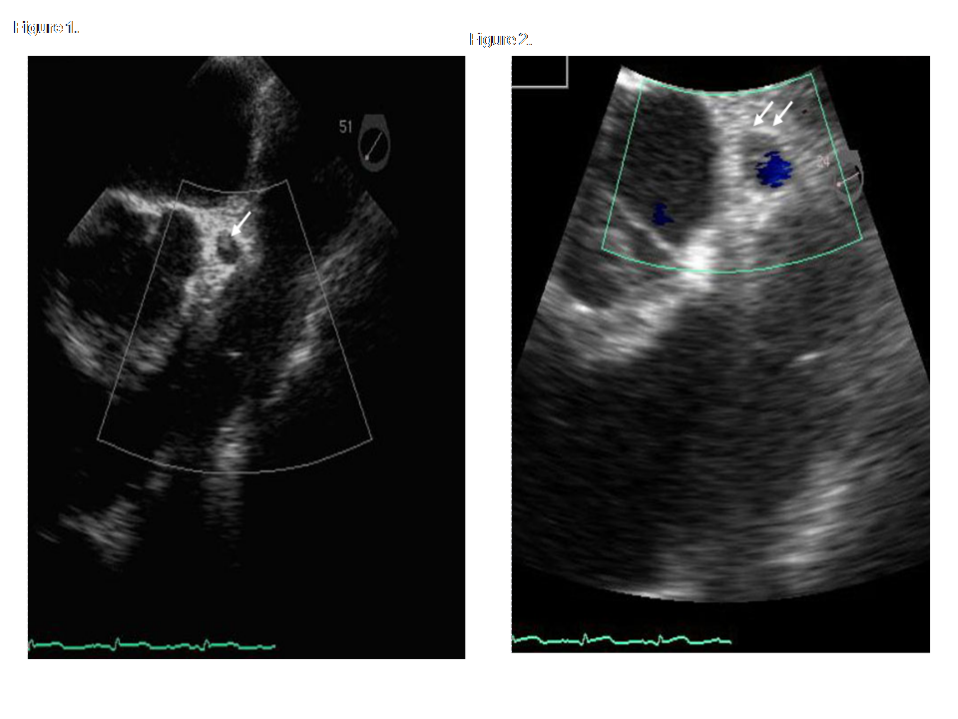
The arrows indicate the echodensity noted in the left main coronary artery with transesophageal echocardiography. Color Doppler flow Interrogation of the left main coronary artery indicate a significant stenosis due to this echodensity (left panel).
